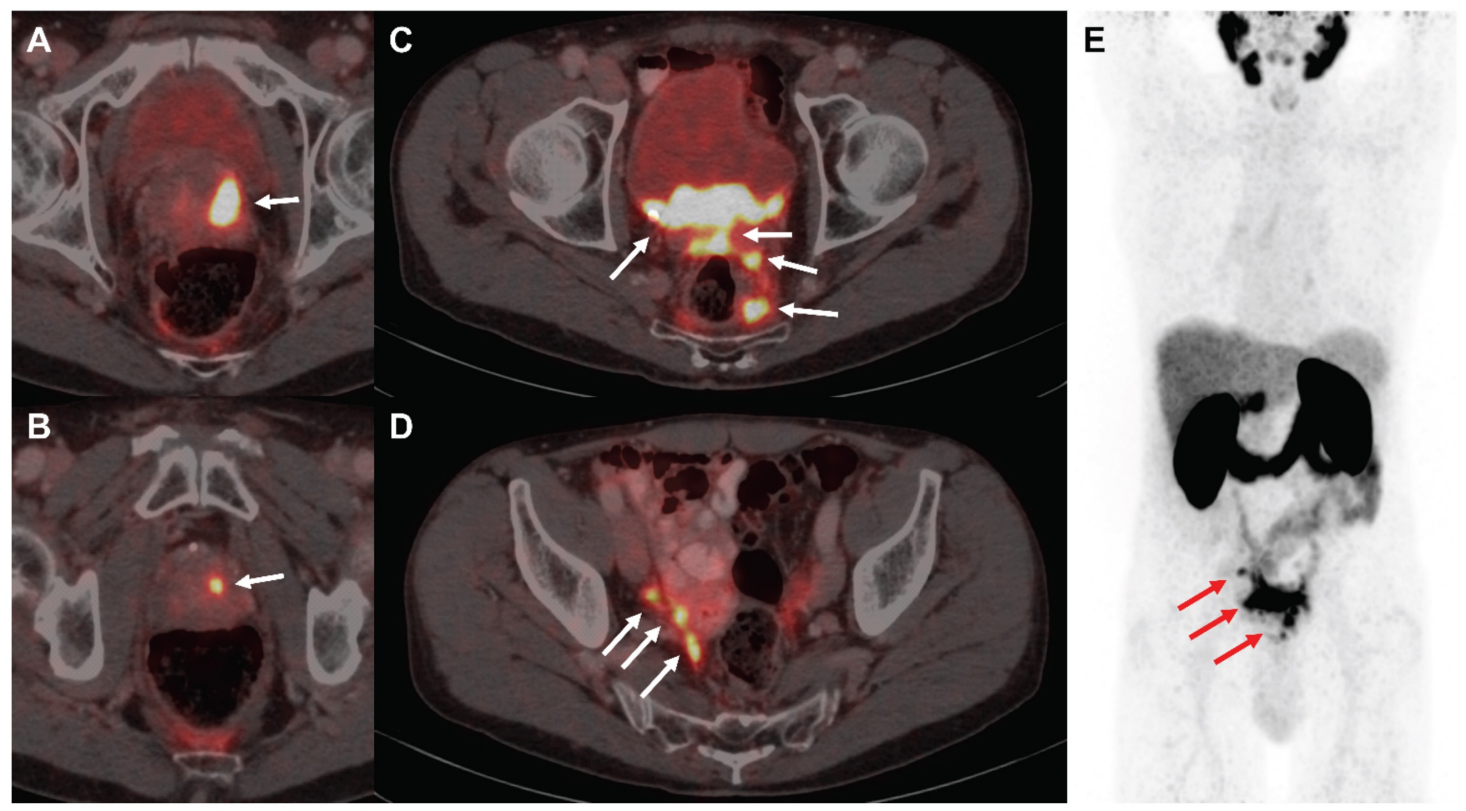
[^68^Ga]Ga-PSMA-11 PET/CT images of the exemplified patient undergoing EBRT followed by PSMA-RLT for locally advanced PC. The patient suffered from multifocal prostate carcinoma (A, B), multiple pelvic nodular tumor lesions with bladder infiltration (C) and lymph node and possible peritoneal metastases in the right internal iliac artery region (D) in PSMA-PET/CT imaging, as indicated by white arrows, respectively. Maximum intensity projection (E) demonstrates absence of organ metastasis, tumor lesions from A-D are depicted using red arrows. Abbreviations: EBRT: external beam radiotherapy; PSMA-PET/CT: prostate-specific membrane antigen-positron emission tomography/ computed tomography; PSMA-RLT: prostate-specific membrane antigen-targeted radioligand therapy.
